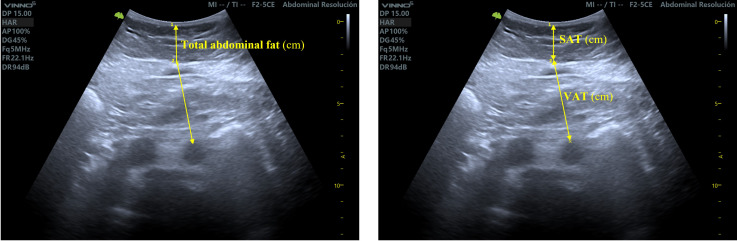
Ultrasound assessment of abdominal fat distribution. SAT, Subcutaneous Adipose Tissue; VAT, Visceral Adipose Tissue.
